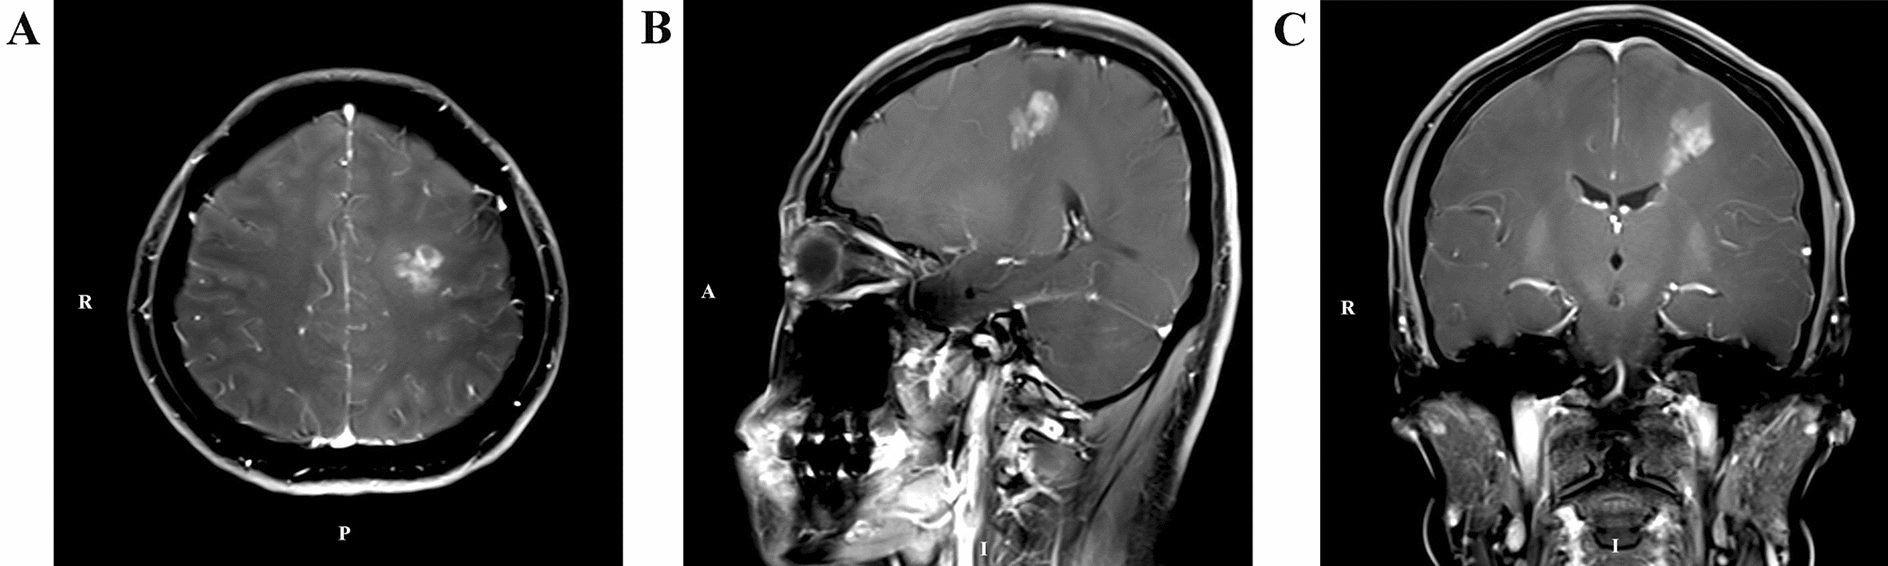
Before surgery, contrast-enhanced T1-weighted magnetic resonance imaging showed a lesion within the left centrum semiovale, with apparently inhomogeneous enhancement and an irregular shape. Edema showed no enhancement. A Axial view. B Sagittal view. C Coronal view
